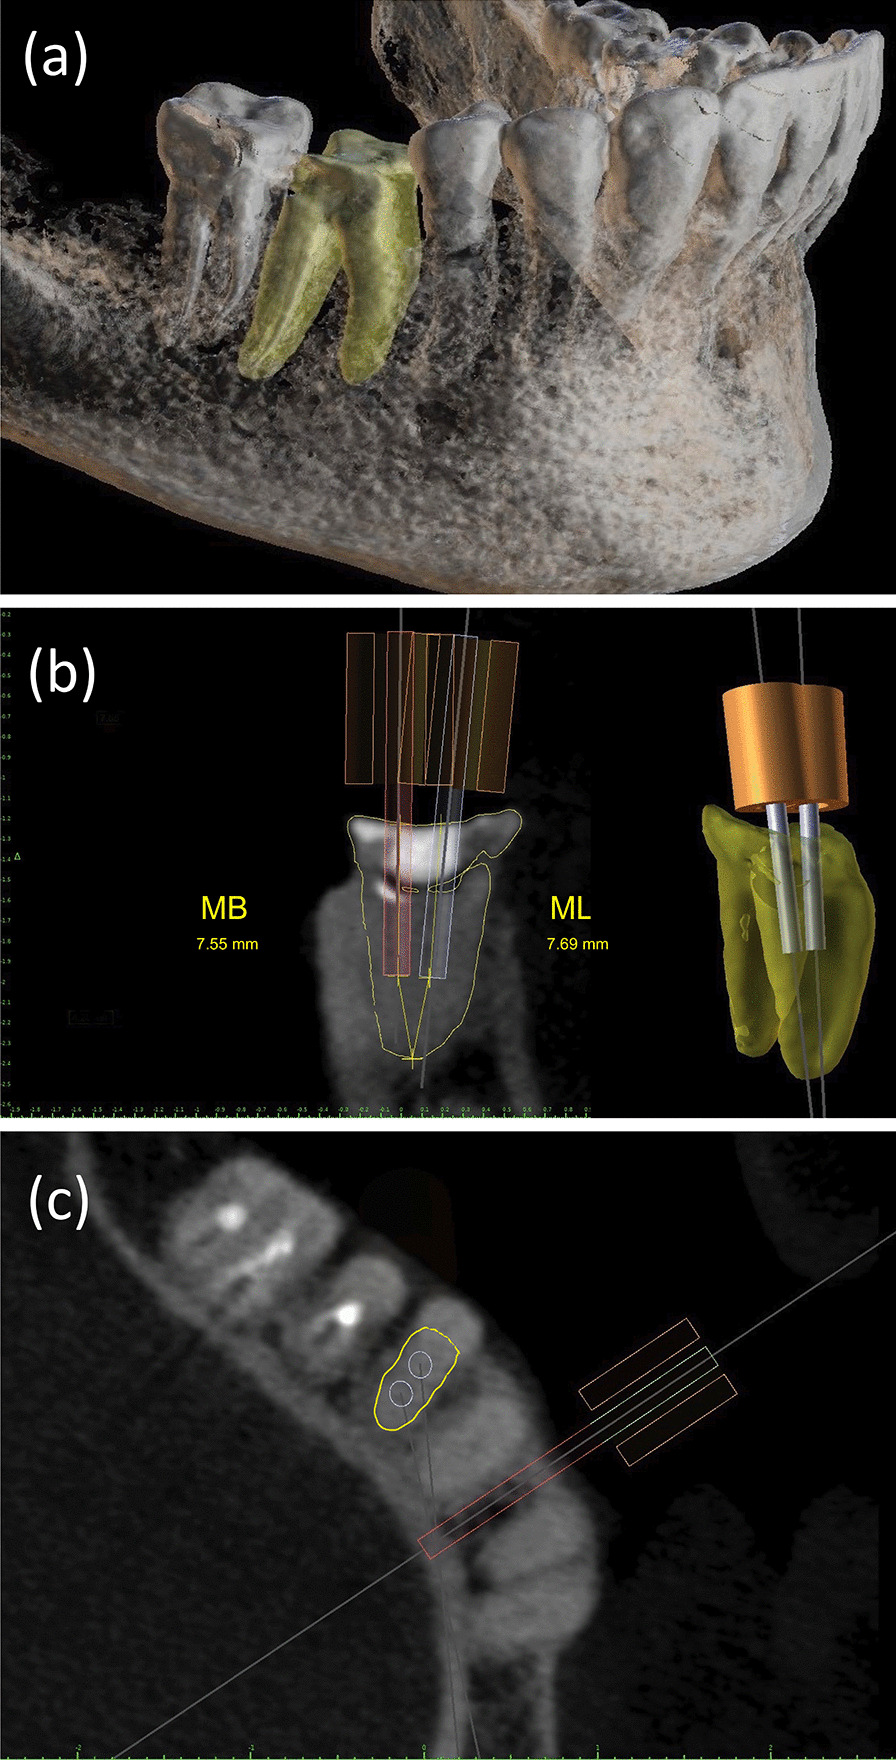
The DICOM data is segmented in an STL file for the 3D reconstruction of the mandible; the mandibular first molar with dystrophic calcification is in yellow (a). The CBCT image shows a buccolingual view of the obliterated mesial root with the virtual drills and respective virtual sleeves positioned in the access pathway (b). MB is the mesiobuccal, and ML is the mesiolingual root canal, respectively. The image also shows an estimated length of the obliterations from the flor of the pulp chamber to the remaining lumens (b). The axial view shows the virtual sleeve and drill for the fixation pin (c)
